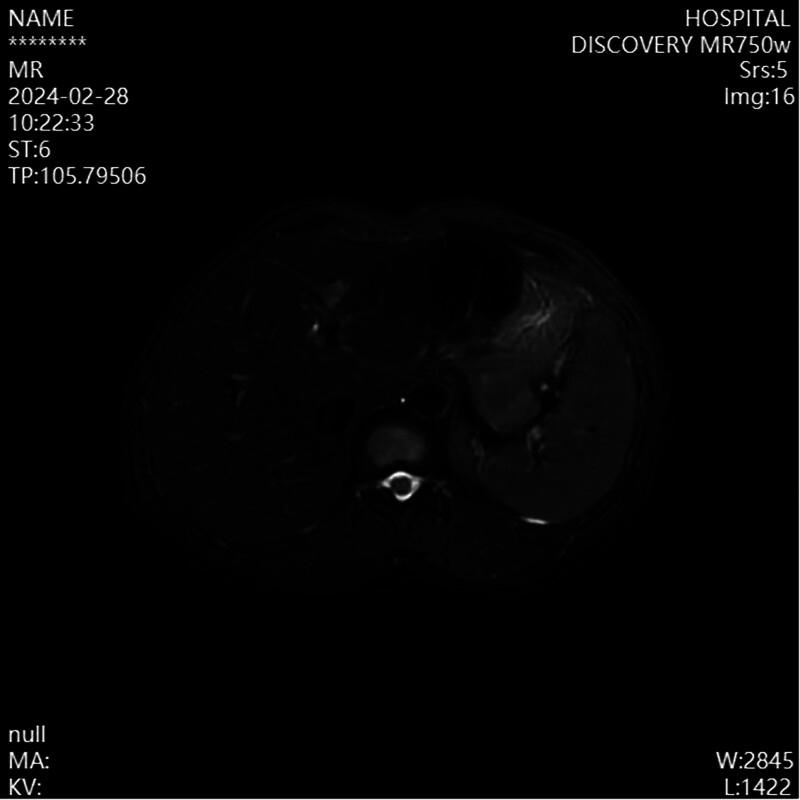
Enhanced MRI of the upper abdomen shows: the liver appears mildly irregular, the spleen is enlarged, with its lower edge significantly extending below the lower margin of the liver; the splenic vein is wide, and tortuous vascular shadows are seen at the splenic hilum. MRI = magnetic resonance imaging.
